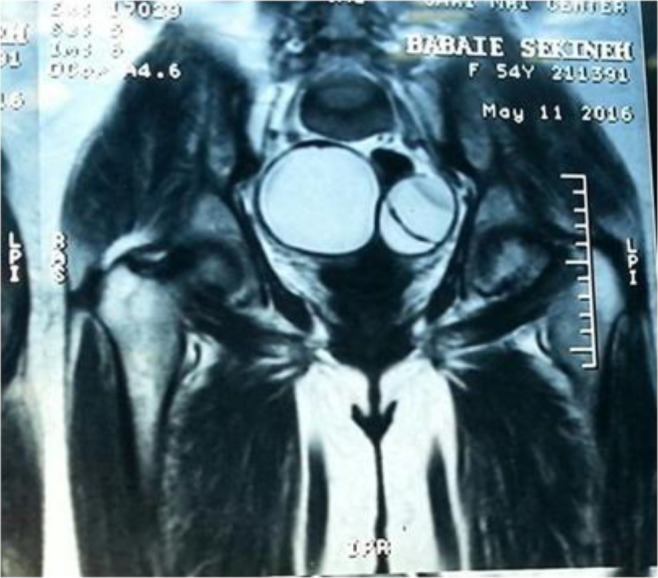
PELVIC MRI, coronal T2 view shows an 80*50 thin layer cyst in right ovarian and a multiloculated 75*40mm cyst in left ovary
